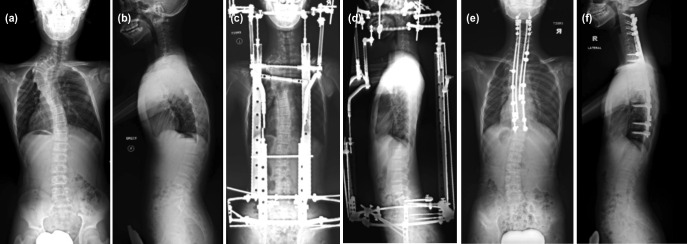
Plain radiographs (posteroanterior and lateral view) of Case 2: (a, b) before operation, (c, d) while on halo-pelvic traction, and (e, f) after single-stage posterior spinal fusion.
